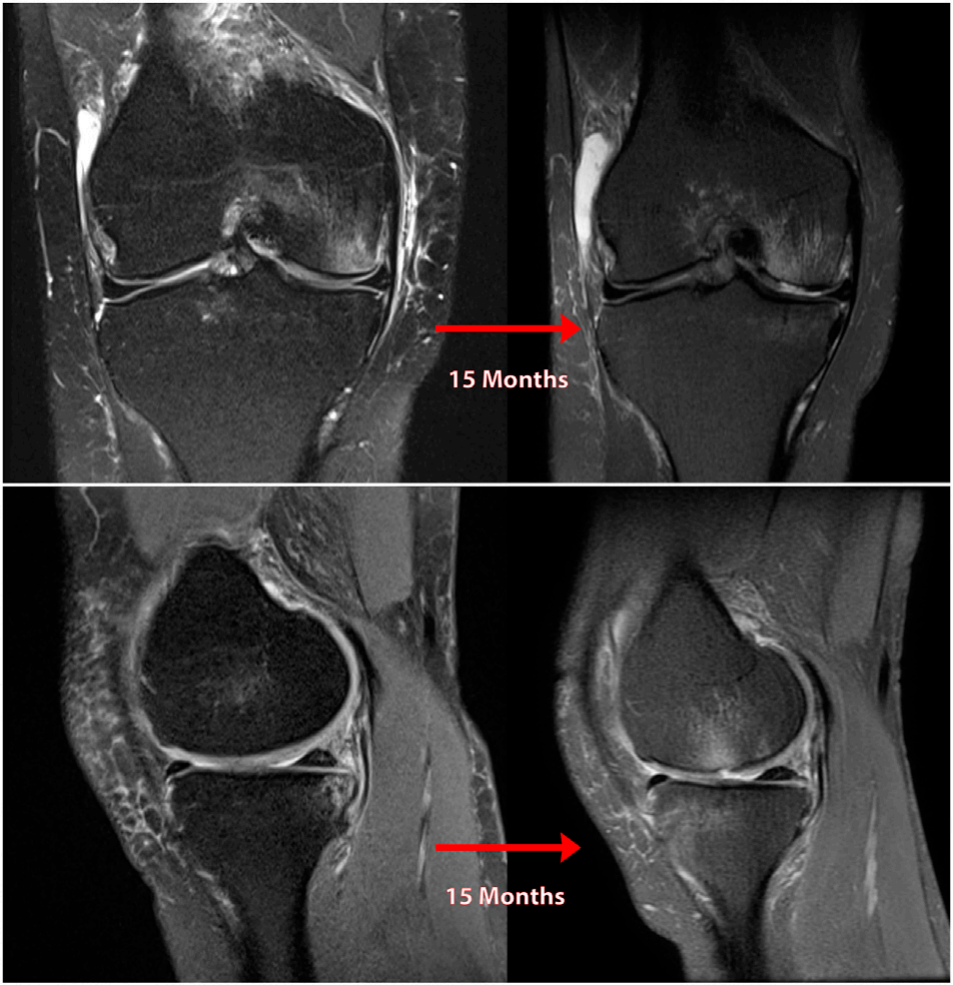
Coronal (A) and sagittal (B) MR images of the knee of natural history patient #5 showing progression of osteoarthritis over 5 months following neglected medial meniscal root tear (right knee).
